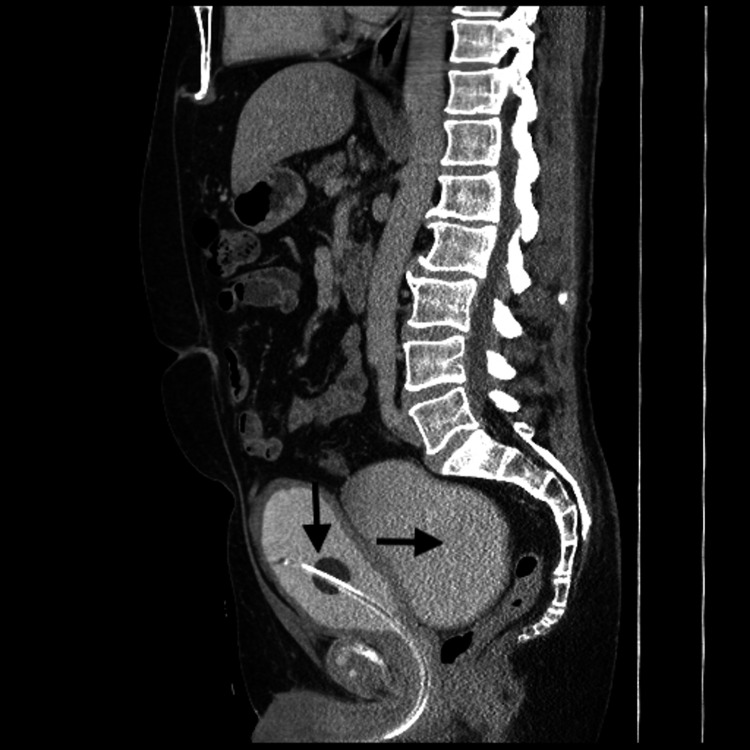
Sagittal view of CT abdomen and pelvis with contrast showing a large posterior bladder diverticulum (horizontal arrow) with a catheter balloon noted in the bladder (vertical arrow)
